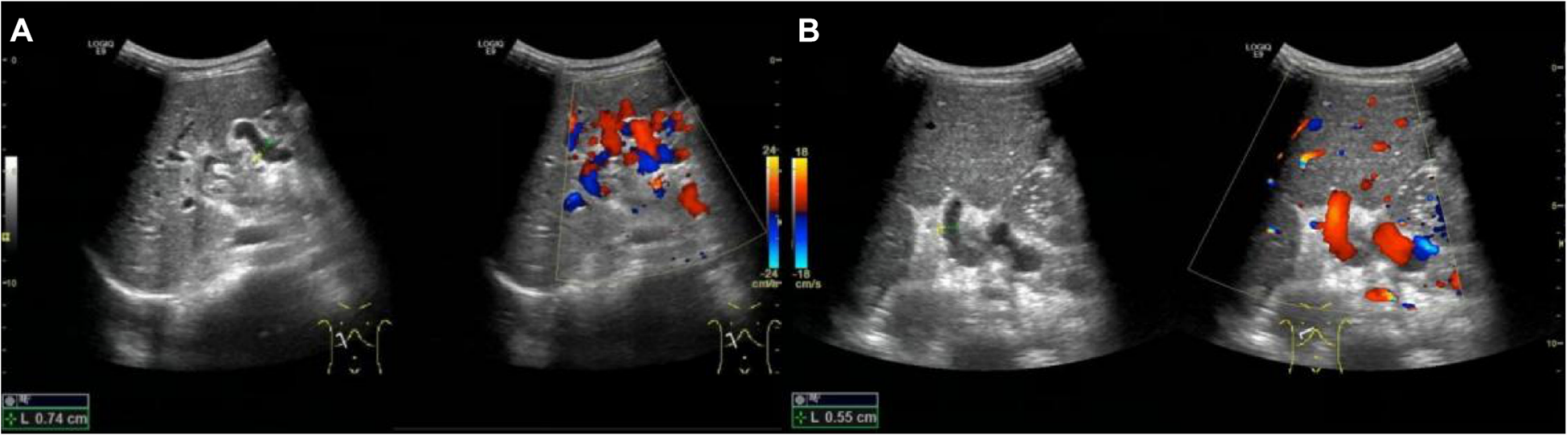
Rich blood flow signals and good patency in the bypass vessels on the color Doppler ultrasonography at 7 days after Rex-bypass operation for CTPV. a 7-year-old boy. b 6-year-old boy
